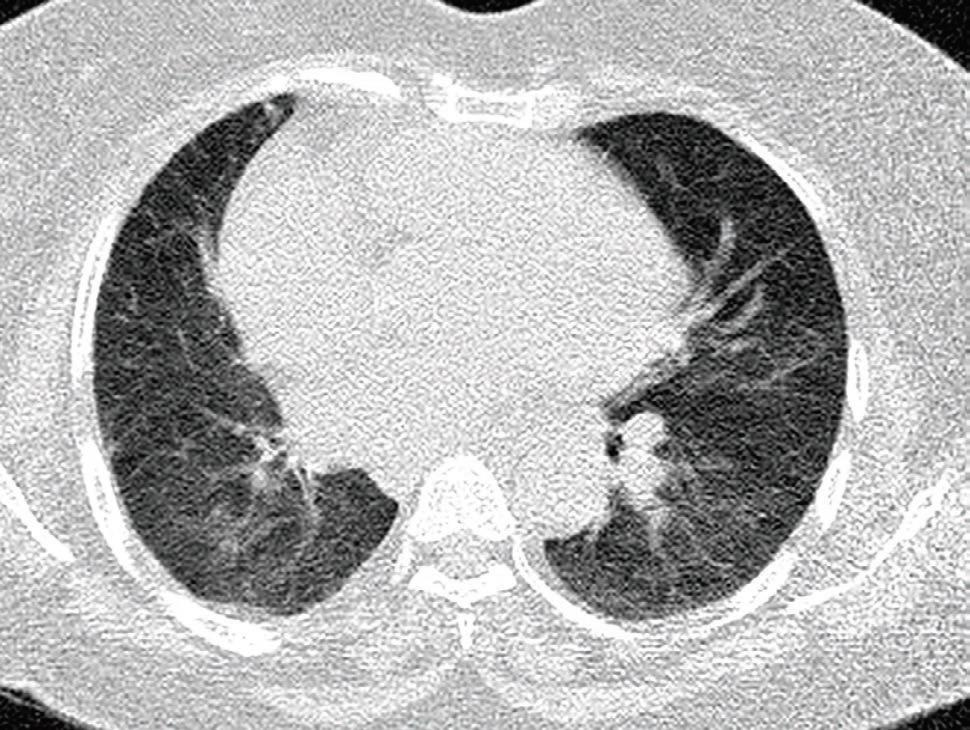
Axial CT images in lung window settings demonstrate mosaic attenuation (patchy regional differences in pulmonary attenuation) and cardiomegaly.
